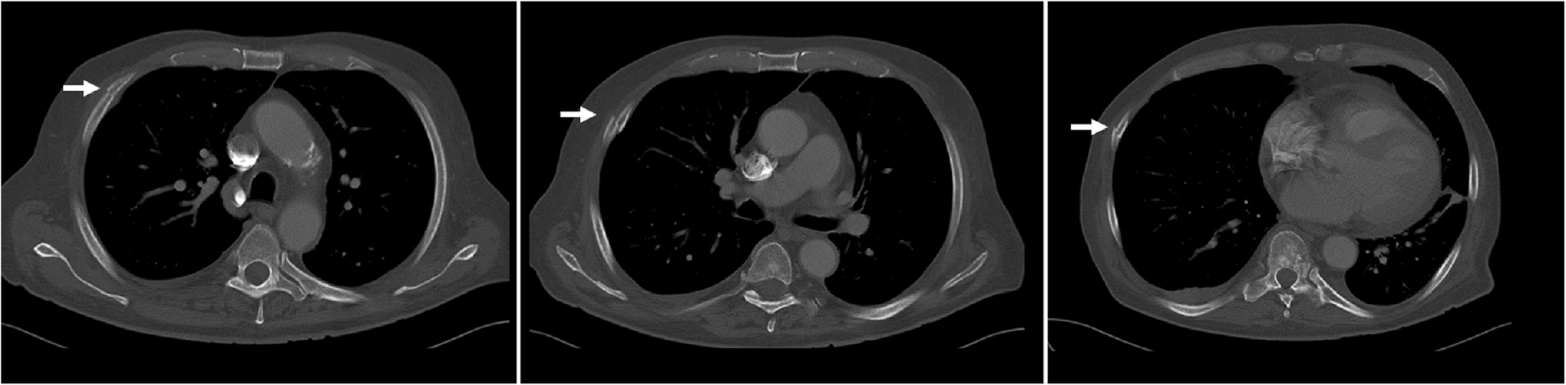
Same patient as in Figures1and2. CT of the chest confirms the fractures of rib IV and V and reveals an additional fracture of rib VII.
